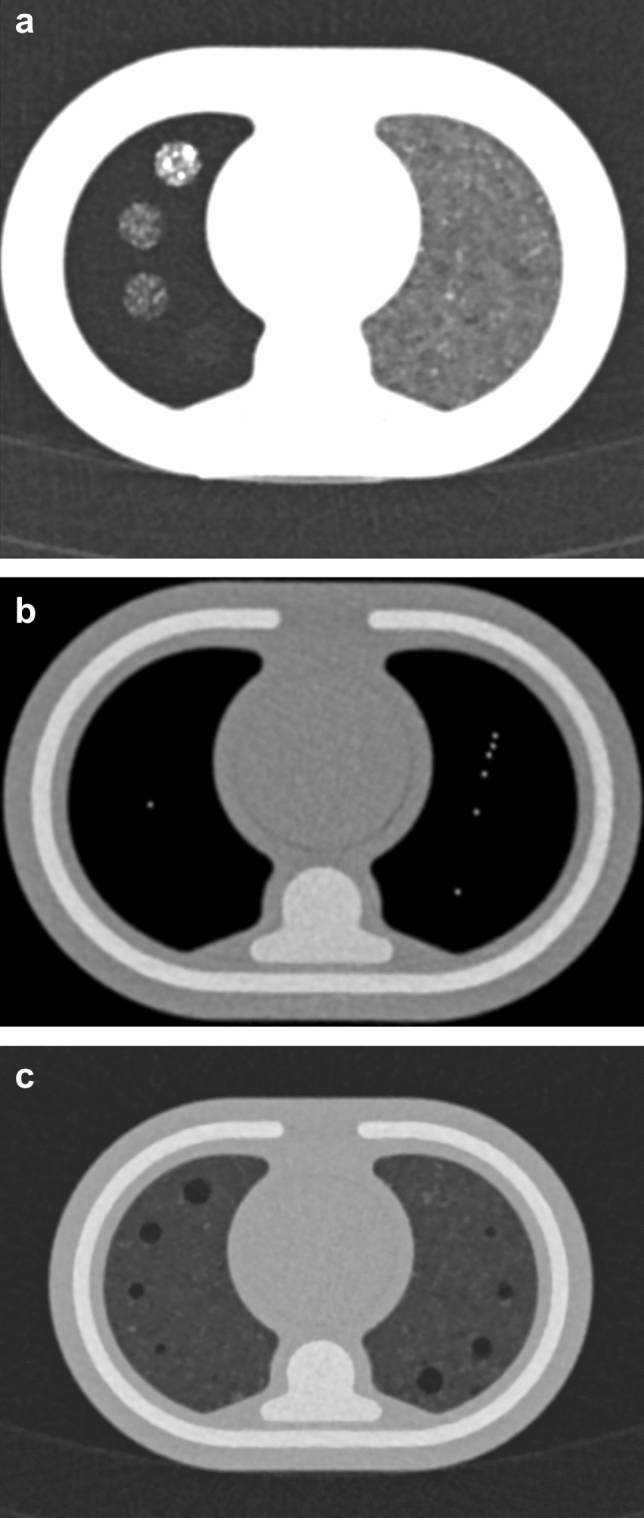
Reconstructed axial CT slices of image quality slices 6, 8 and 9: a Slice 6, the contrast resolution tool showing iodinated contrast levels inside the lung substitute. b Slice 8, the spatial resolution slice, showing thin Nichrome wire point source used to produce the MTF (left), and a 6-point calliper tool (right). c Slice 9, the contrast detail test tool with gradually smaller air gaps
